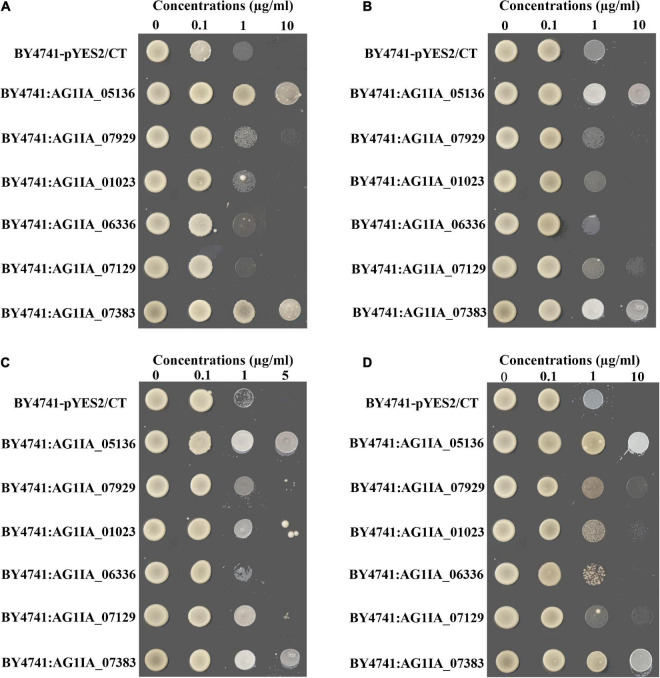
Heterologous expression of the cytochrome P450 genes *AG1IA_05136*, *AG1IA_01023*, *AG1IA_07129*, *AG1IA_07929*, and *AG1IA_06336* and glutathione transferase gene *AG1IA_07383* in a drug-hypersensitive *Saccharomyces cerevisiae* strain BY4741. The sensitivity of the transformants were tested on solid synthetic dextrose minimal agar medium lacking uracil, containing 2% galactose and amended with different concentrations of SYP-14288 (A), fluazinam (B), chlorothalonil (C), and difenoconazole (D). *Saccharomyces cerevisiae* strain BY4741 was transformed with expression vector pYES2/CT (BY4741-pYZS2/CT), with P450 genes *AG1IA_05136* (BY4741:AG1IA_05136), *AG1IA_07929* (BY4741:AG1IA_07929), *AG1IA_01023* (BY4741:AG1IA_01023), *AG1IA_06336* (BY4741:AG1IA_06336), and *AG1IA_07129* (BY4741:AG1IA_07129) and with glutathione S-transferase gene *AG1IA_07383* (BY4741: AG1IA_07383).
